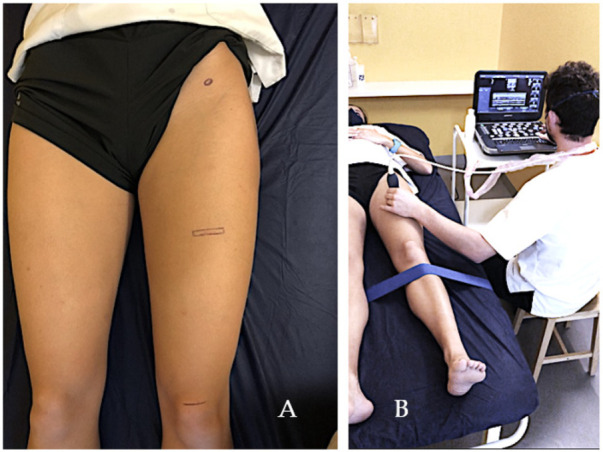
Researcher and patient preparations and positioning. Surface references (A) and ultrasound assessment sampling (B).
